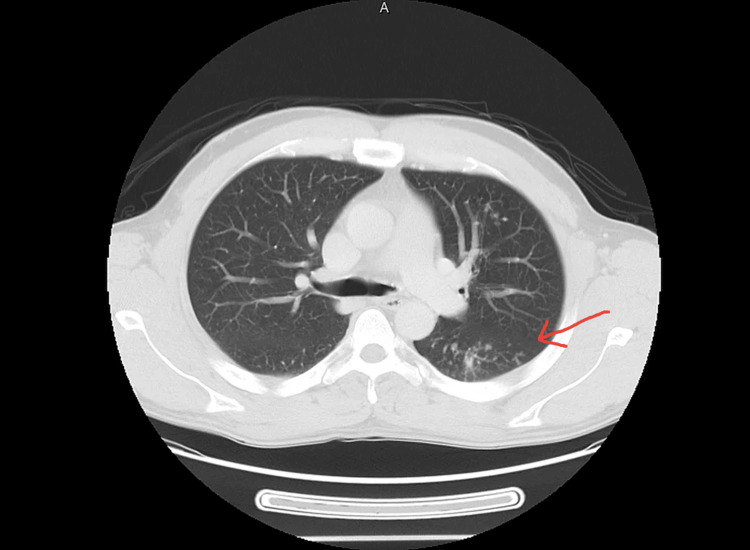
CT scan of the chest Performed in October 2022.
